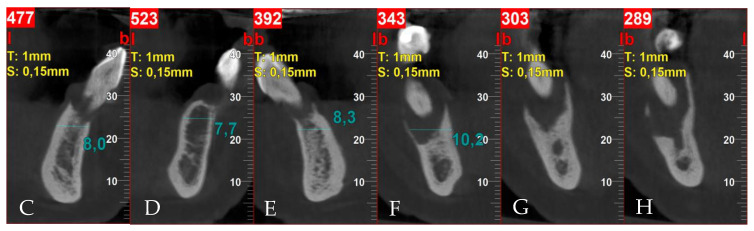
Pre-treatment CBCT: (A) panoramic view; (B) axial view; (C) cross-sections in position of teeth, respectively, 32; (D) 34; (E) 42; (F) 44; (G) 45; (H) 45.
